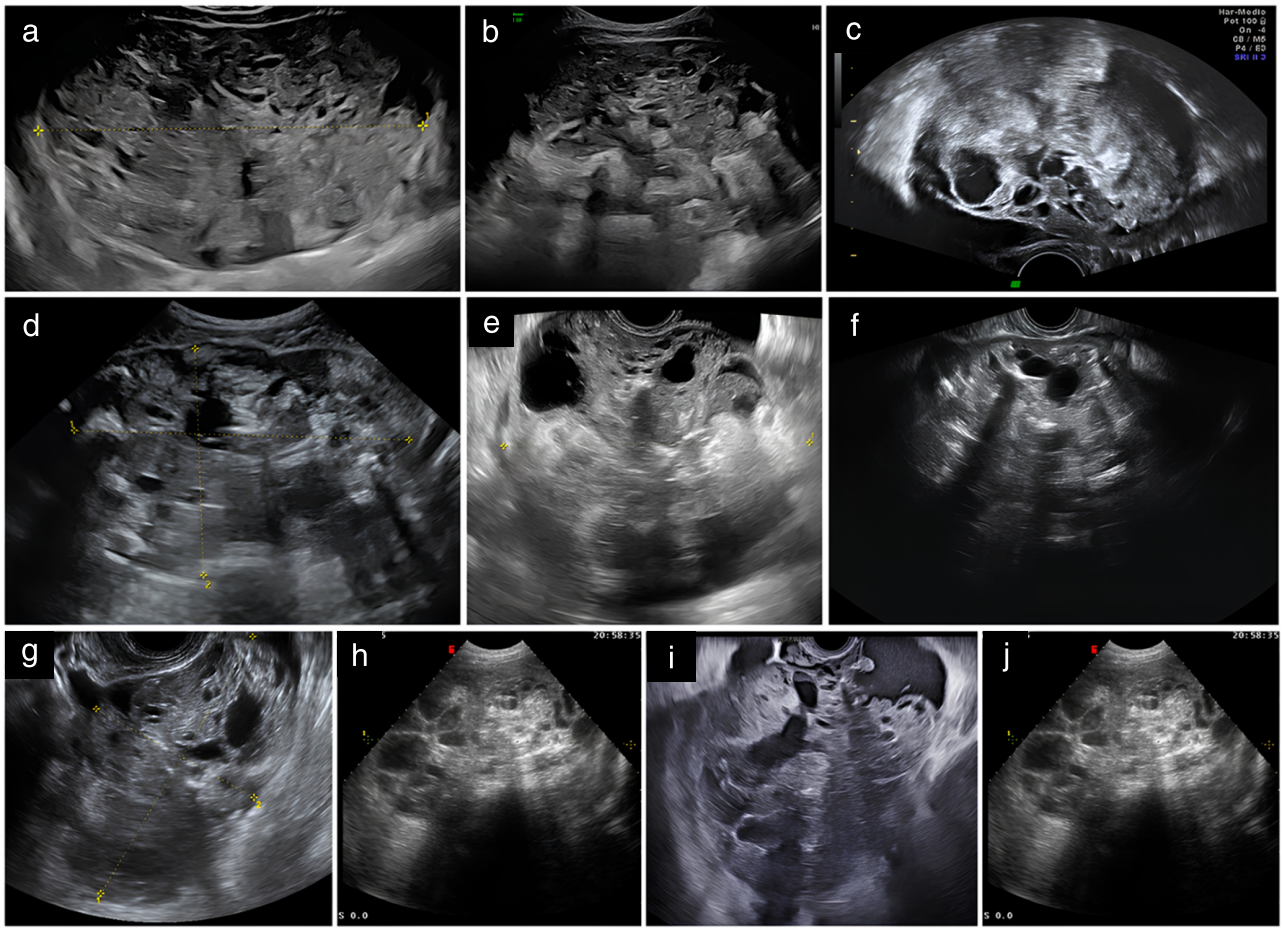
(a–j) Grayscale ultrasound images of 10 ovarian immature teratomas. Using pattern recognition, these tumors were described as solid tumors (lesions consisting of ≥ 80% solid tissue). They all show pathognomonic echogenicity of solid tissue, with hyperechogenic areas, cysts and shadowing.
